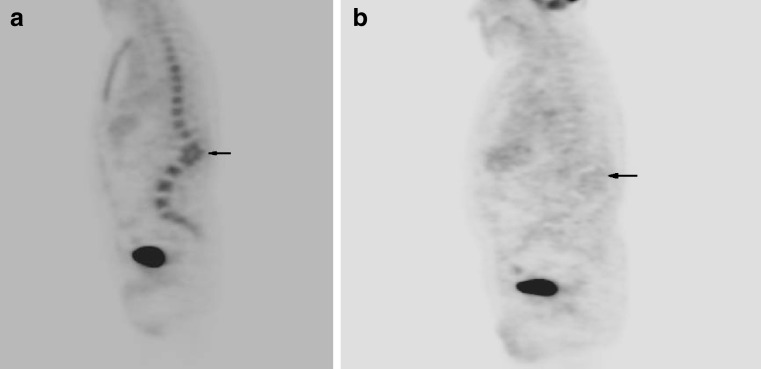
a False-positive compression fracture in a patient on bone marrow-stimulating agents. Sagittal FDG-PET image demonstrates diffuse increased radiotracer activity throughout the spine. Note the compression fractures with focal kyphotic angulation at L1–L2 (*arrow*). The patient was thought to have diffuse metastatic disease with malignant compression fracture. b Sagittal FDG-PET image performed 2 months later demonstrates resolution of diffuse radiotracer uptake. Kyphotic angulation from compression fractures remains (*arrow*). The patient was off bone marrow-stimulating agents for 6 weeks at the time of the study
